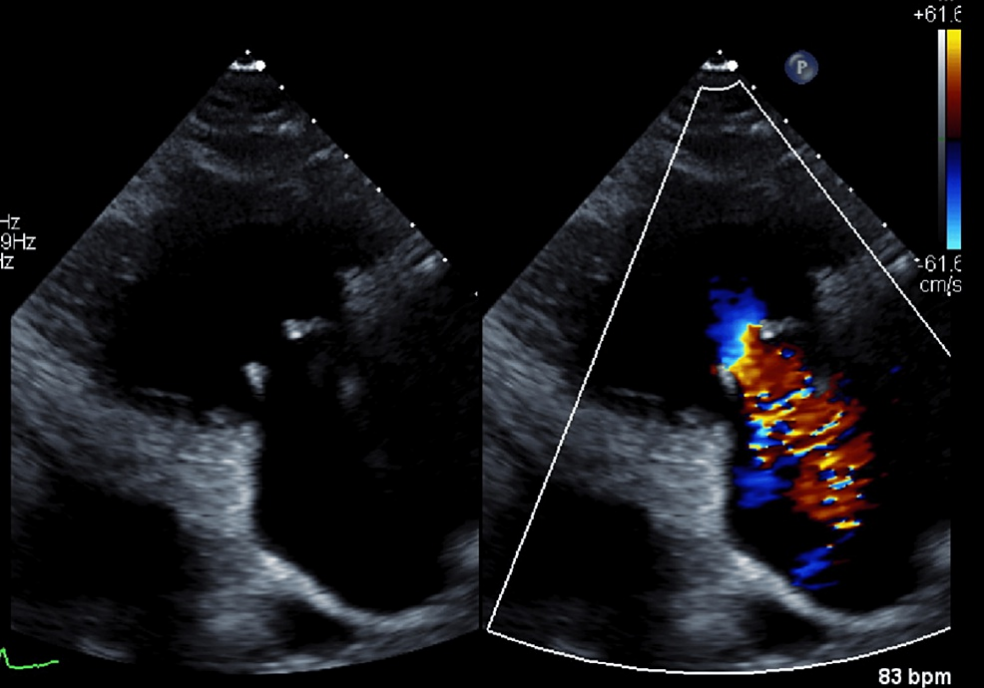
A color Doppler ultrasound of the tricuspid valve, with a jet of blood flowing downward (away from the transducer), indicated tricuspid regurgitation.
